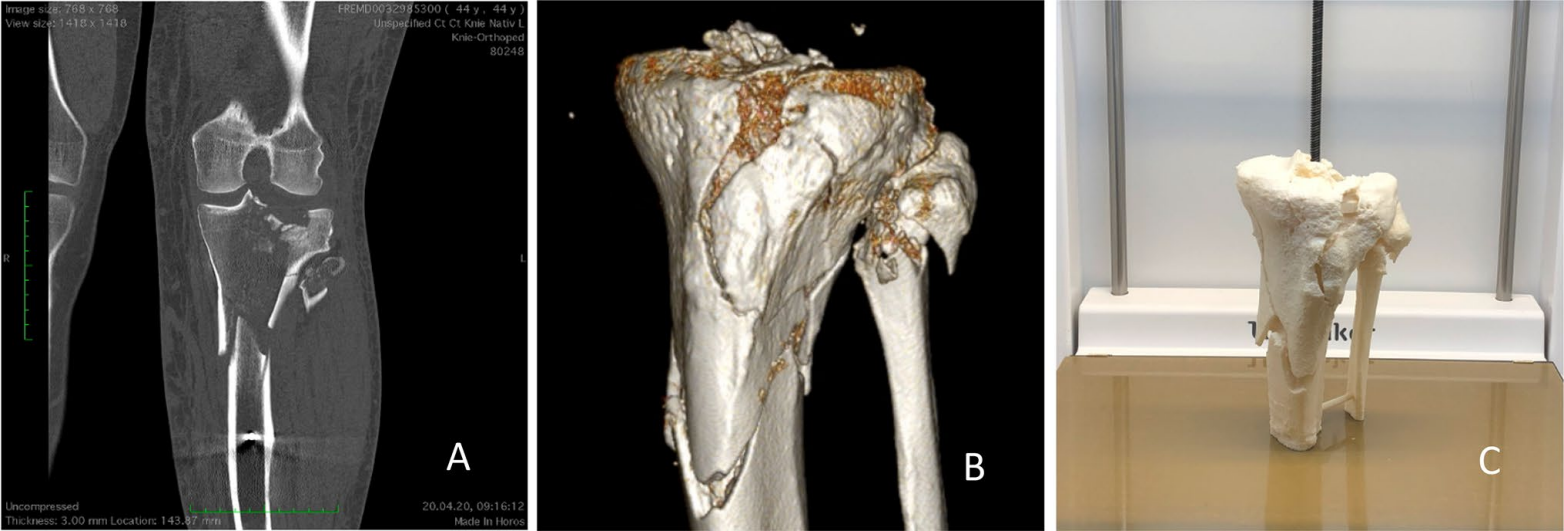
Tibial plateau fracture shown in CT (A), 3D reconstruction (B) and 3D-printed fracture model (C) imaging modalities
